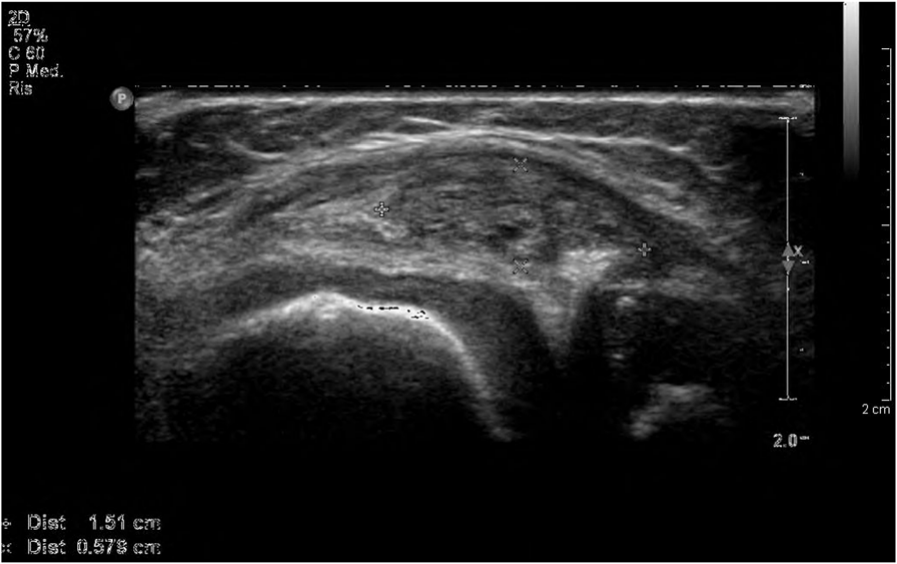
Ultrasonography of patient 4 shows a hyperechogenic mass in the medial part of the right knee
